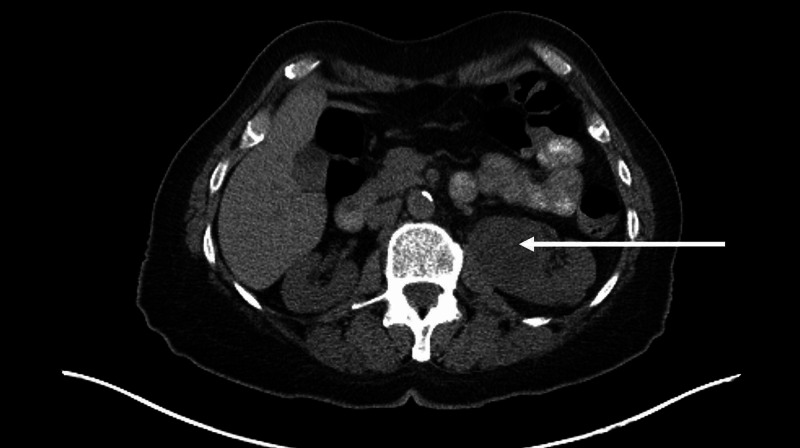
Outside imaging of left renal mass (CT scan) Arrow: Indicates the left renal mass
